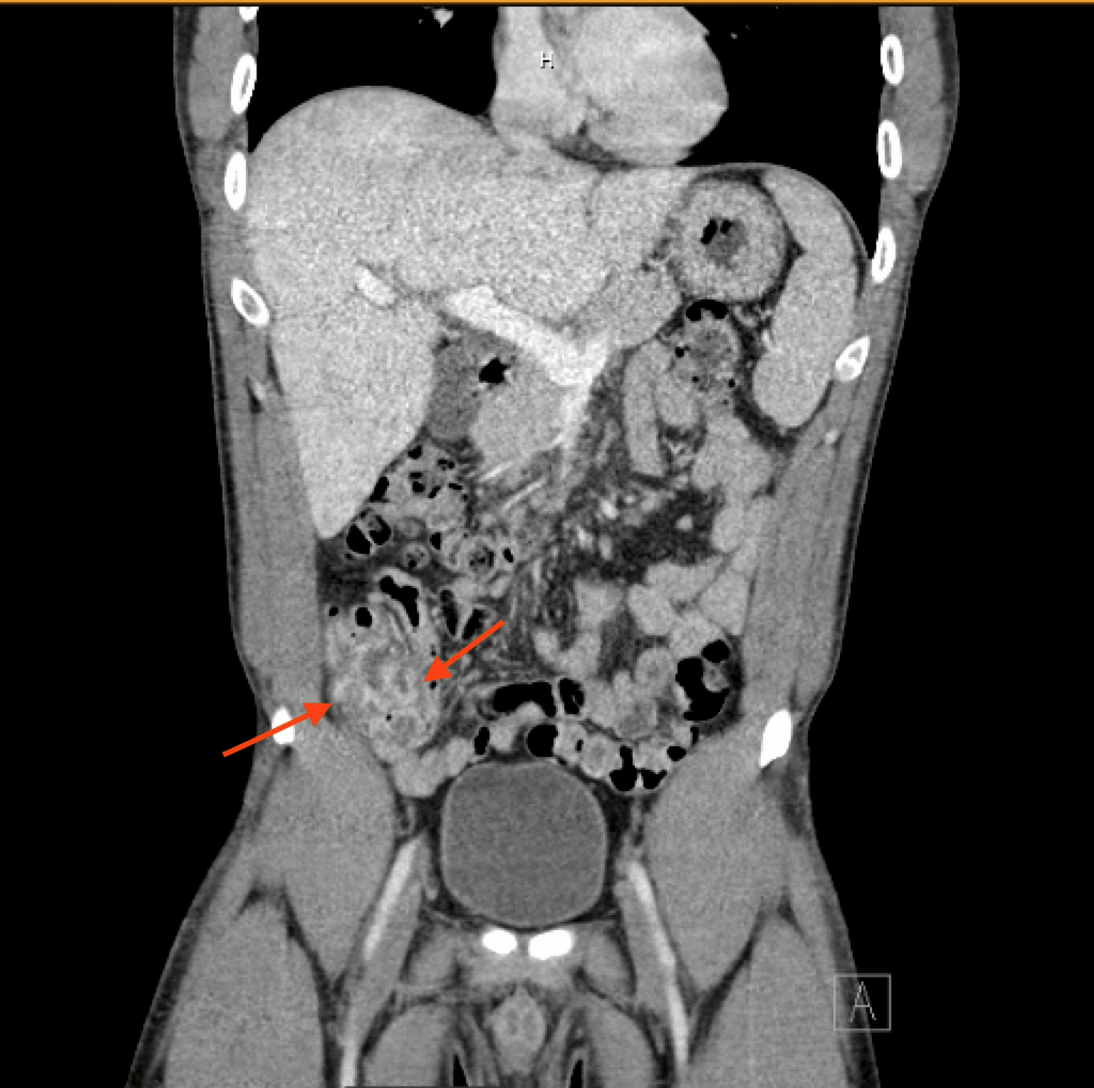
CT abdomen and pelvis showing mild colitis Image of CT abdomen and pelvis showing mildly thickened cecum/ascending colon walls (marked with red arrows), suggestive of colitis
